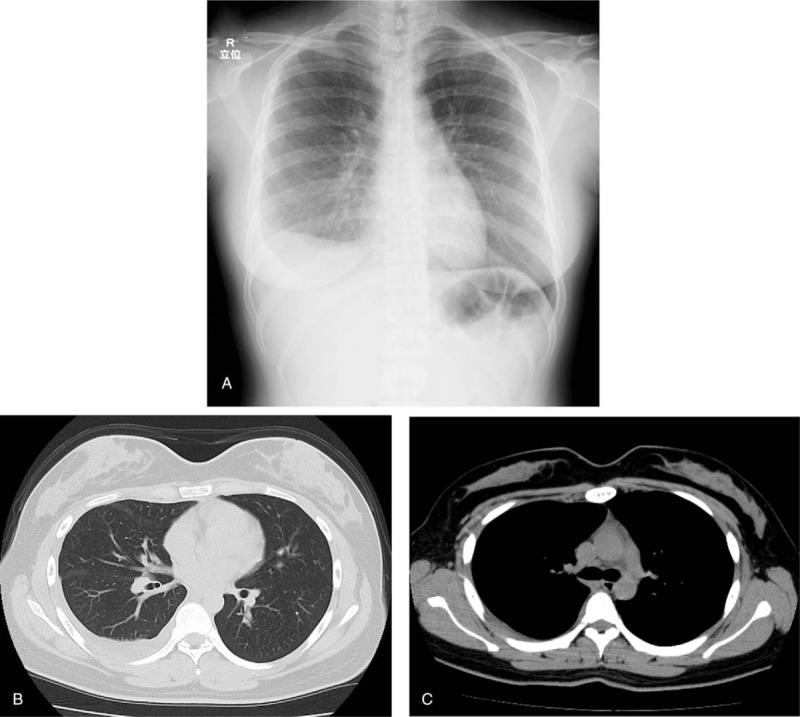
(A) Chest X-ray revealed right pleural effusion. (B), (C) Chest plain computed tomography (CT) revealed right pleural effusion but no intrapulmonary lesion or significant enlargement of the hilar or mediastinal lymph node.
